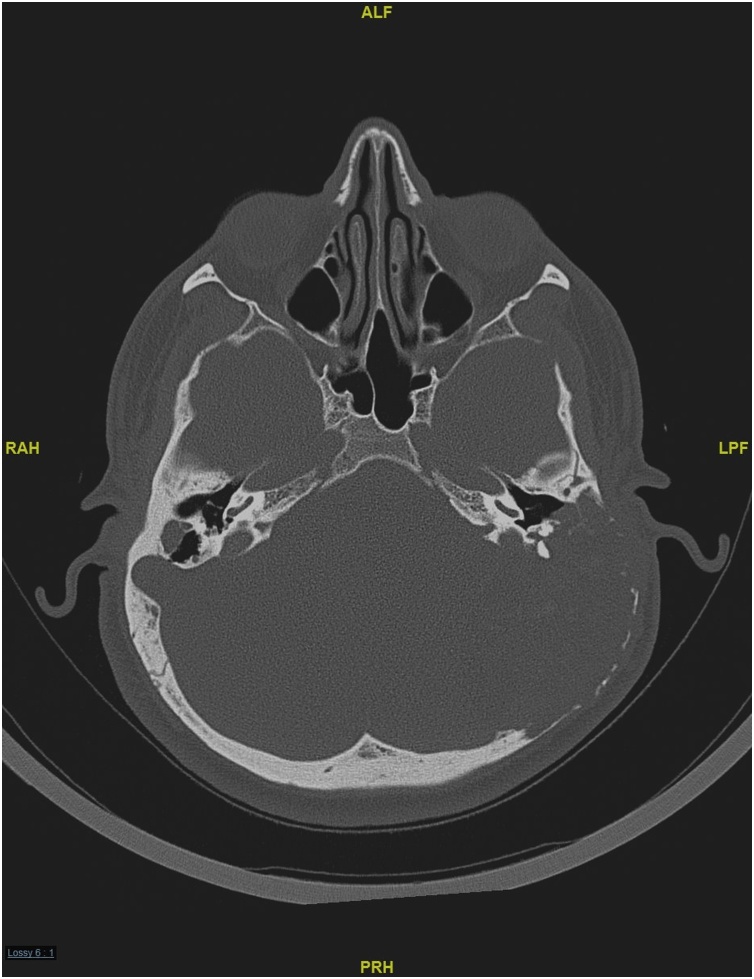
Computed tomography (CT) scan of the temporal bone showing a large, expansile, heterogenous mass, centered in the left tympanomastoid region. The mass was bulging into the external auditory canal, middle ear cavity, and extending posteriorly to temporal and occipital region. Smaller lesion is seen on the right side within the mastoid cavity.
